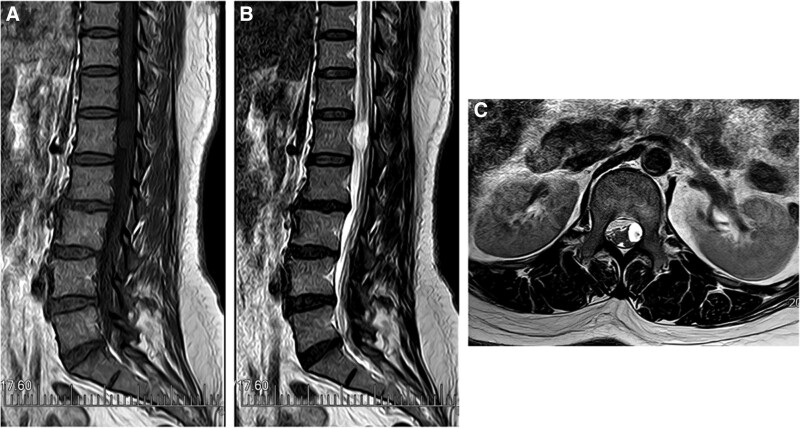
(A) Preoperatively sagittal T1-weighted lumbar spine MRI scan. (B) Preoperatively sagittal T2-weighted lumbar spine MRI scan. (C) Preoperatively axial T2-weighted lumbar spine MRI scan. MRI = magnetic resonance imaging.
